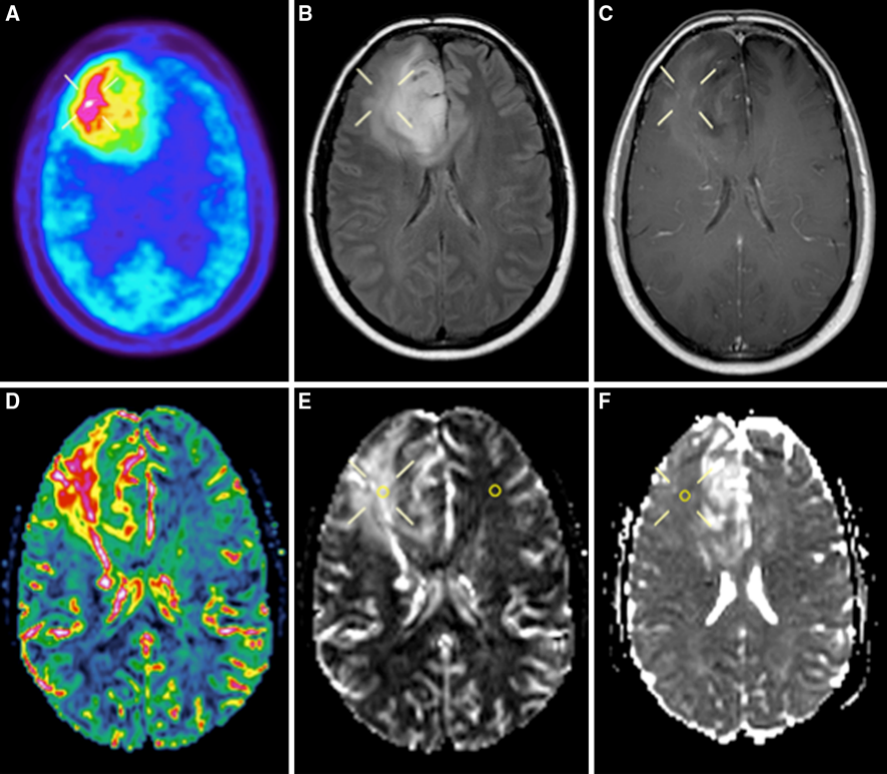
Patient 8. Preoperative MET PET and MRI of a right-sided frontal oligodendroglioma grade II in a 31-year-old female. a MET PET shows the hotspot region of the tumor. b T2-weighted FLAIR MRI shows a high signal intensity tumor. c T1-weighted contrast-enhanced MRI shows minimal contrast enhancement in the tumor area. d DSC perfusion MRI with rCBV color map shows high perfusion in the tumor area. e DSC perfusion MRI with rCBV *grey-scale* map shows high perfusion in the region corresponding to the PET hotspot. The ROIs in the tumor and in the contralateral normal appearing white matter are marked. f dMRI where the MD_min_ value in the region corresponding to the PET hotspot is lower than MD in the medial portion of the tumor (but minimally increased compared to normal appearing contralateral white matter)
